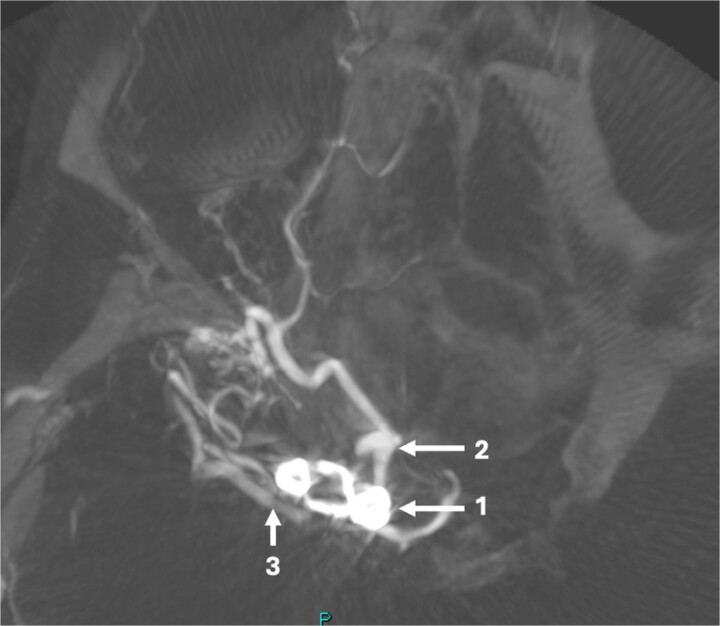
Axial post-operative CT angiogram demonstrating the (1) encircling clip occlusion of the terminal internal carotid artery aneurysm with a relatively normal calibre of (2) A1 and, (3) M1.
